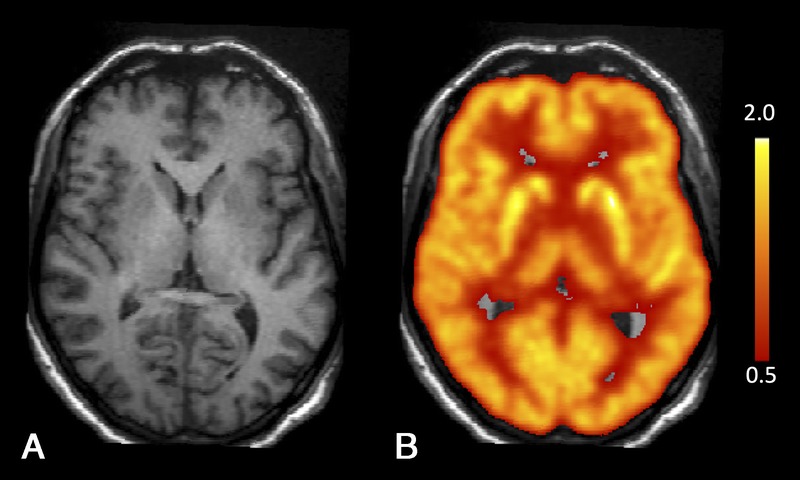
A, T1-weighted 1.5 T brain magnetic resonance imaging showing no abnormal findings. B, Normal brain glucose metabolism on [^18^F]FDG positron emission tomography. The figure shows [^18^F]FDG uptake normalized to the average uptake of the whole brain (red-yellow scale).
